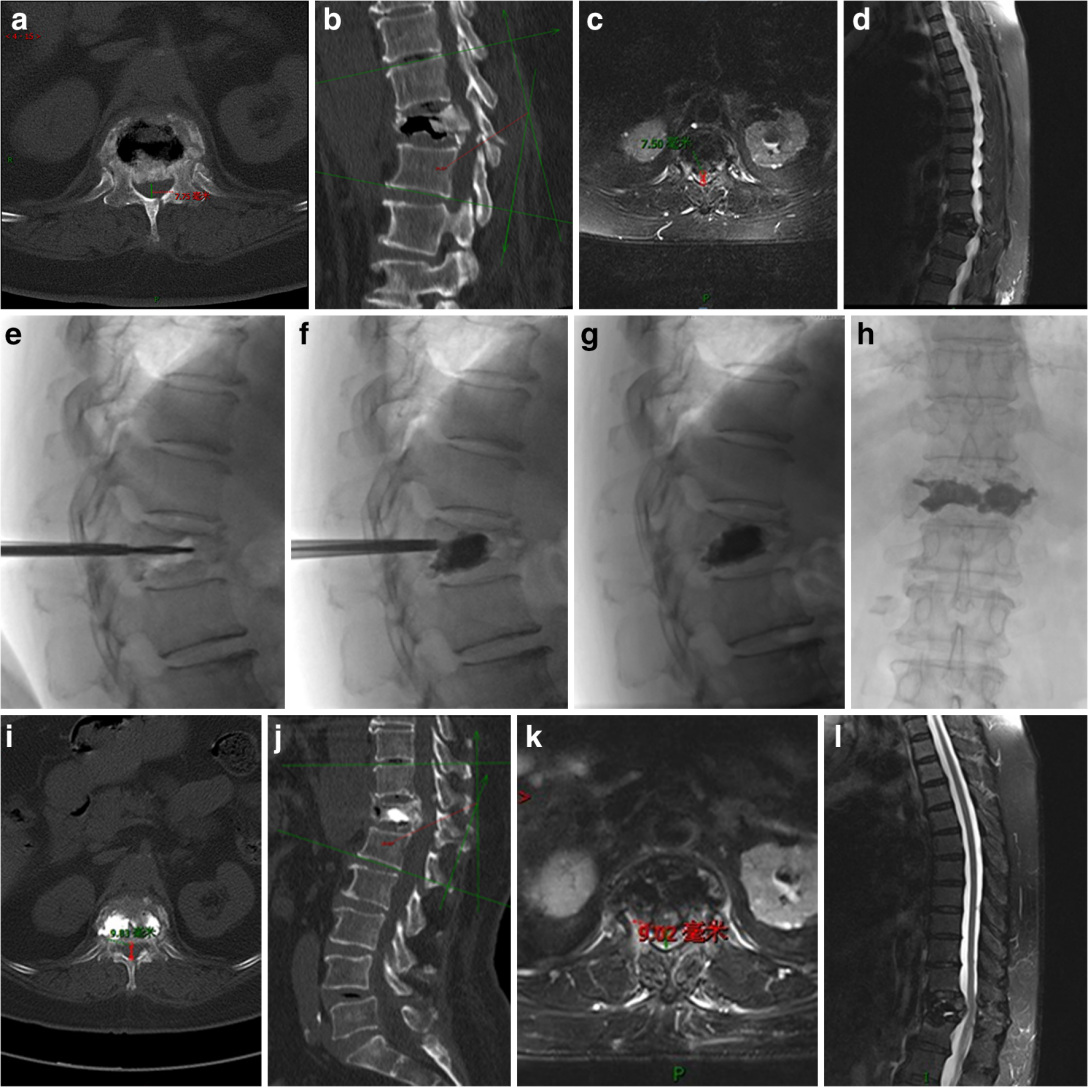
A 79-year-old female patient admitted because of chest and low back pain for more than 1 month. CT and MRI showed Kummell’s disease phase III with spinal canal compression but without neurological symptoms. Before operation (a–d): MRI and CT showed *T*_12_ level with an intravertebral vacuum cleft. Vertebral body and adnexal bone were interrupted continuously with vertebral wedge (compression 1/2). The posterior margin of the vertebral body bulges backwards, the anteroposterior diameter (7.5–7.75 mm) of the spinal canal was narrowed and Cobb’s angle was 24.22°. During operation (e–h): anteroposterior and lateral view showed the placement of the bone-filling mesh container. The vertebral body was punctured through the bilateral vertebral pedicle approach, the mesh bag implanted, and the bone cement filled well without leakage. After operation (i–l): MRI showed the T_12_ vertebra filled with bone cement. Vertebral body angle was improved and the compression reduced to 1/3. CT showed the widened diameter (9.02–9.83 mm) of the spinal canal, Cobb’s angle was 19.93°, and bone cement filled well without leakage
